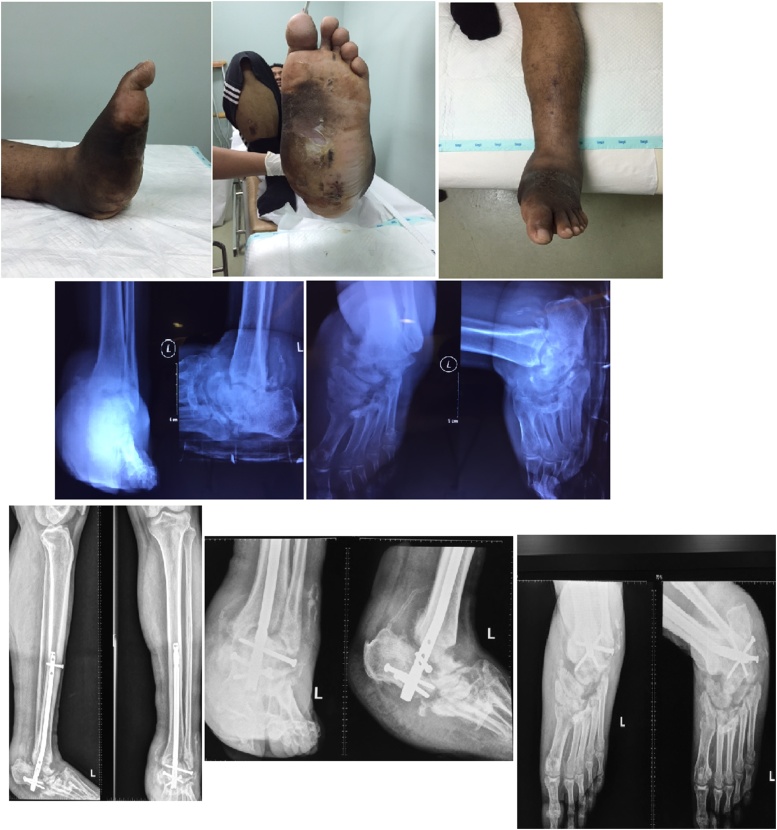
Physical examination and radiography of second case. (A) Physical examintions and (B) radiography showed rocker bottom deformity of the left foot. (C) Radiograpy of the left foot after CCT arthrodesis using ETN.
